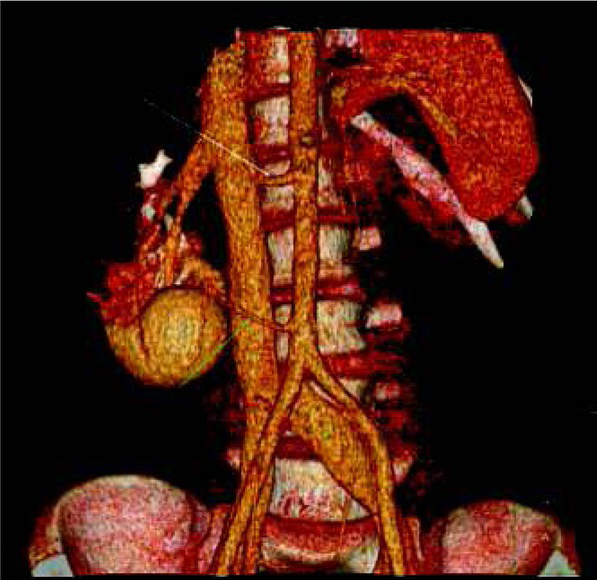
Volume reconstruction of CT performed in a 14-year-old boy with agenesis of the left kidney, after traumatic injury of the right kidney. One-phase split-bolus CT shows two renal arteries (arrows) with amputation of the upper artery (white arrow) and no enhancement of corresponding upper and middle part of the kidney. There were no signs of injury of the collecting system. Ryc. 4. Rekonstrukcja objętościowa TK wykonanej u 14-letniego chłopca z agenezją nerki lewej, po urazie nerki prawej. Jednofazowa TK z podzielonym bolusem środka kontrastowego wykazała obecność dwóch tętnic nerkowych (strzałki) oraz brak zakontrastowania dystalnego odcinka górnej tętnicy (biała strzałka) i odpowiadającej jej środkowej i górnej części miąższu nerki. Badanie nie wykazało zmian urazowych układu zbiorczego nerki.
